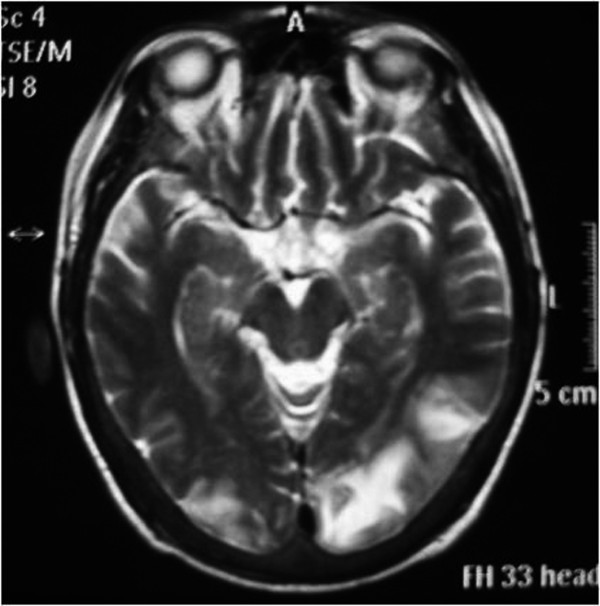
Brain MRI T2 Bilateral parieto-occipital hyperintese lesion.
